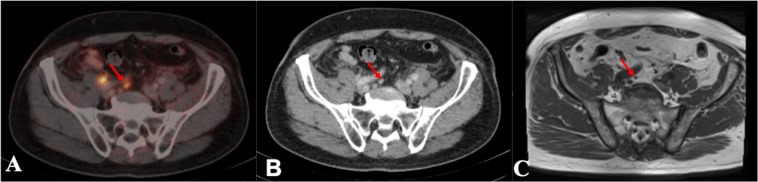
Example of a lymph node smaller than 10 mm in ^68^Gallium-Prostate specific membrane antigen PET-CT compared to T1 MRI. This figure shows a suspicious lymph node smaller than 10 mm diameter. The lymph node is visualized in corresponding axial plane slices using CT, ^68^Gallium-Prostate specific membrane antigen PET-CT and T1 MRI from left to right. The suspected lymph node is located at the right common iliac artery and is challenging to detect in T1 MRI. The lymph node is highlighted through red arrows. (A) Lymph node depicted in CT, (B) Lymph node depicted in ^68^Gallium-Prostate specific membrane antigen PET-CT, (C) Lymph node depicted in the T1 sequence in MRI.
